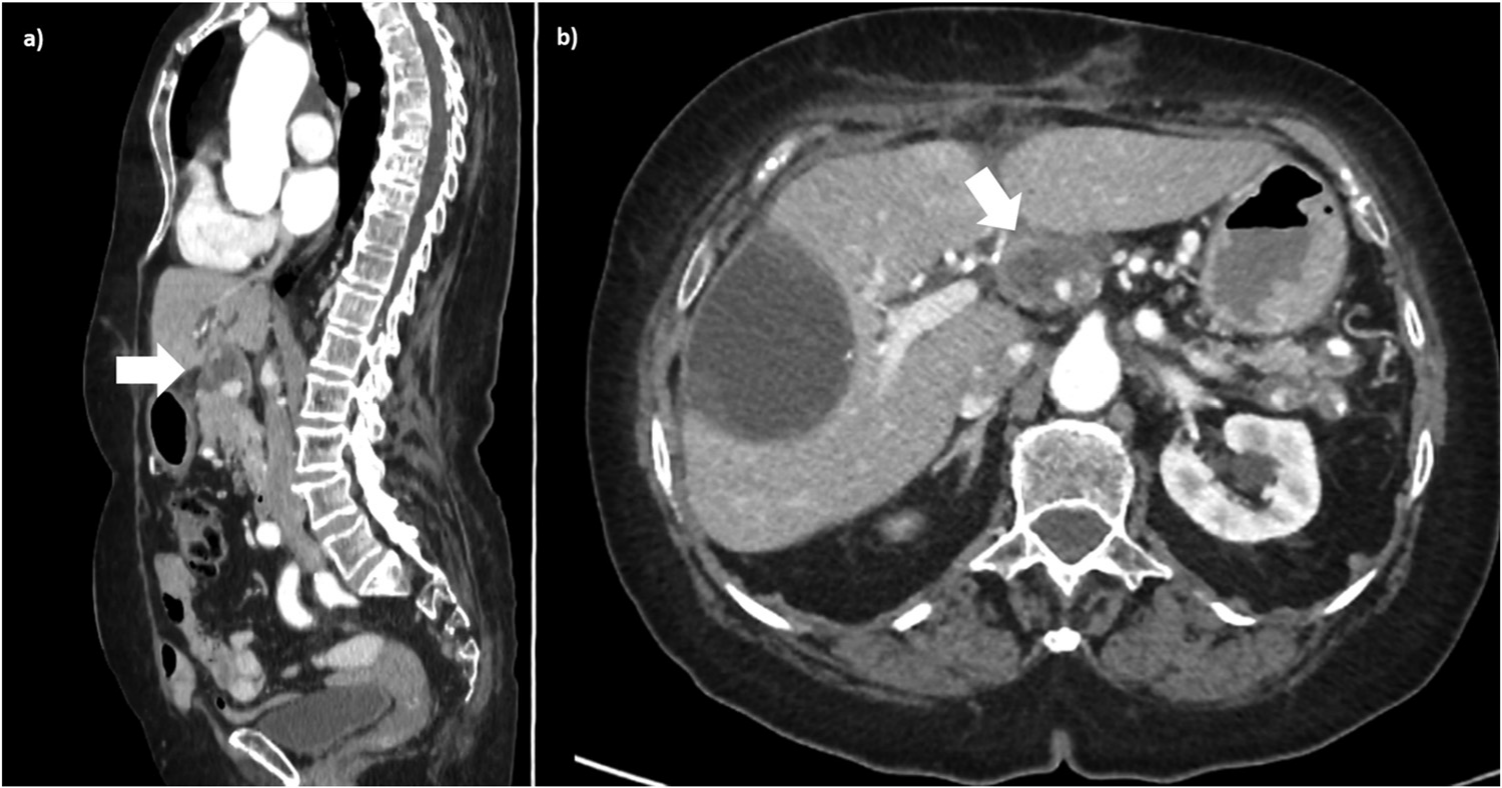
6 months postoperative follow-up. CT scan during arterial phase of the contrast study, in MPR sagittal plan (a) and in axial plane (b): the arrow shows complete thrombosed CHA aneurysm and his vascular exclusion.
